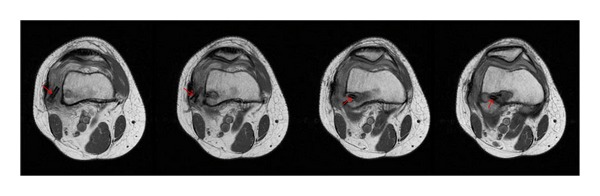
Axial PD fat suppressed T2-weighted images of a right knee in a patient with prior anterior cruciate reconstruction demonstrate a fractured Bio-TransFix cross pin (red arrows).
